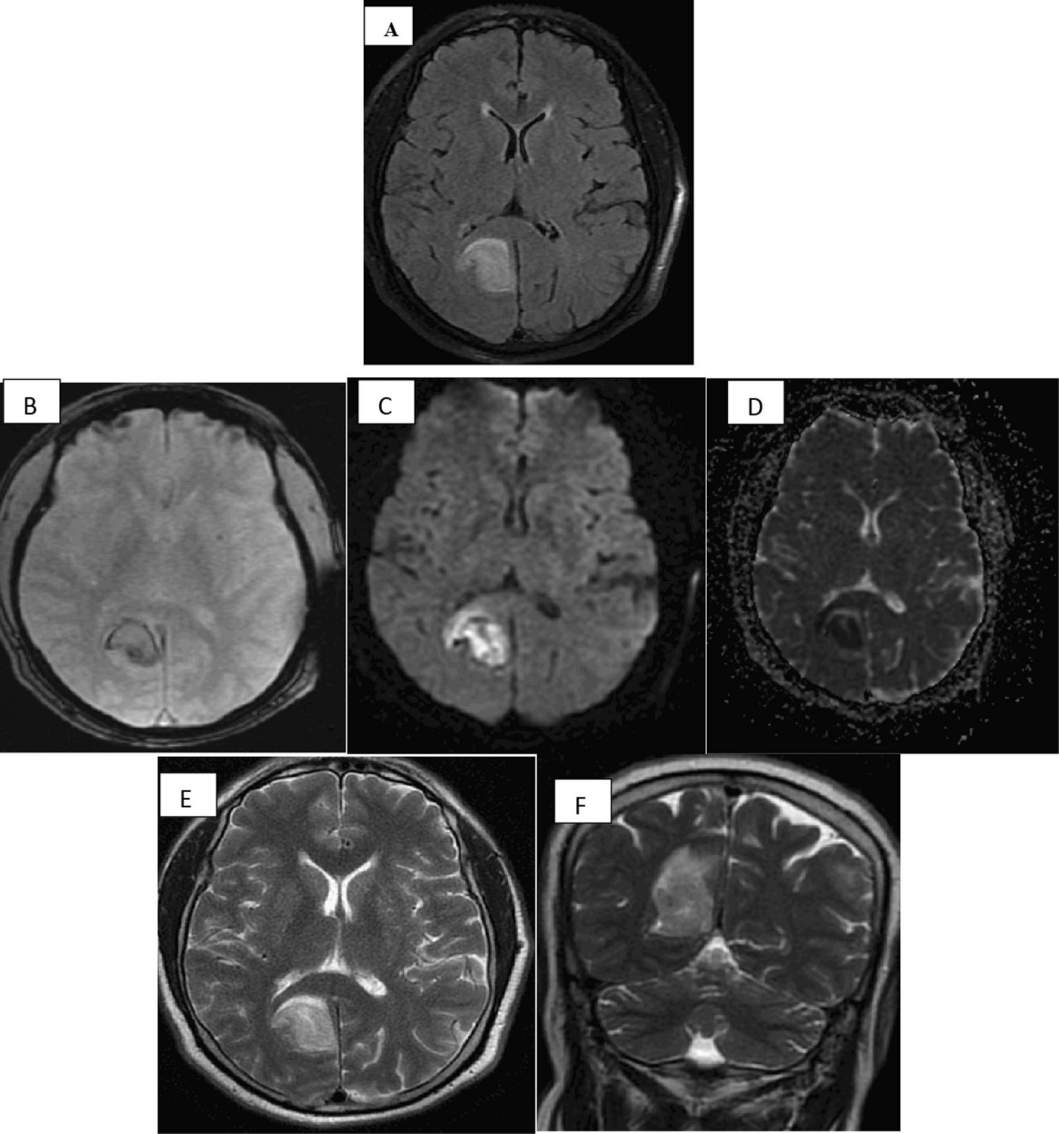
A 58-year-old female with COVID-19 infection confirmed by PCR test, presented with fever, cough, 1 week later, she came with unilateral weakness. Cortical and sub-cortical area of altered signal intensity is seen in the right occipital lobe. A Axial FLAIR showing right occipital area of heterogenous signal intensity. B Axial gradient sequence showing right occipital area of heterogenous signal intensity with rim of low signal intensity. C DWI (*b*-value 1000) showing restricted diffusion. D ADC image, showing suppression of restricted high diffusion signal. E Axial T2WI, F coronal T2WI showing heterogenous signal intensity. Diagnosis: Right posterior cerebral arterial territory recent haemorrhagic infarction
